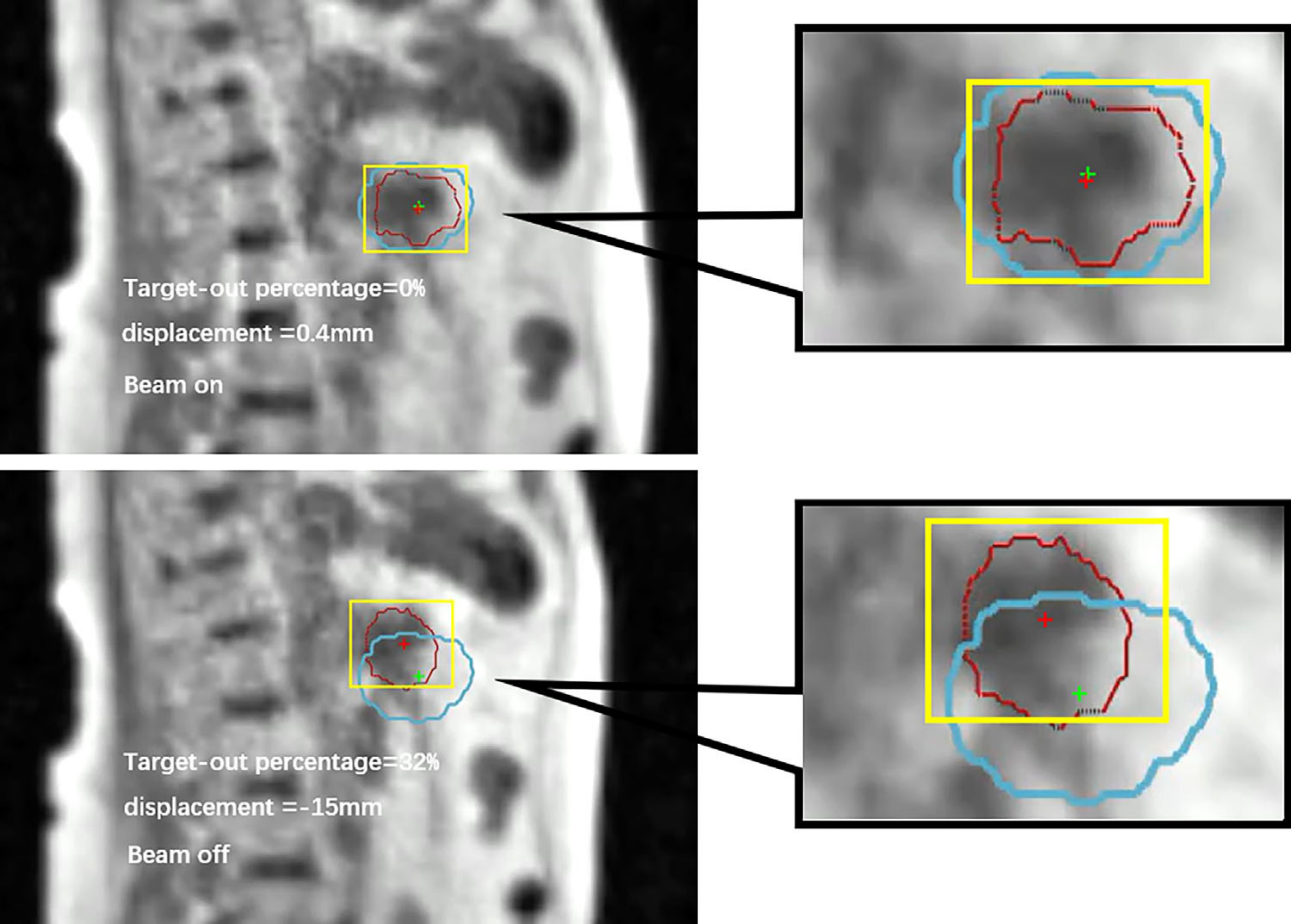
Two cine MRI‐based tumor gating strategies. In the tumor contour‐based gating strategy, the radiation beam is on when GTV (red contour) is inside the gating boundary (blue contour). Radiation is turned off when the target‐out percentage exceeds a predefined threshold, i.e., 5%. In the tumor displacement‐based gating strategy, the centroid of the tracking area (yellow box) is defined as the tracking position (red cross), and radiation is delivered only when the tracking position displacement is less than a predefined gating threshold, i.e., 4 mm. The green cross indicates the baseline position. The right panels show the enlarged area surrounding the tracking area.
